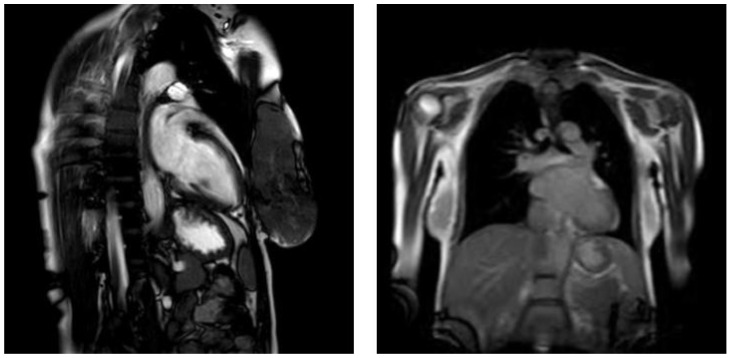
Cardiac MRI of patient reported the enlargement of left ventricle with hypokinesis of the mid-inferior and inferolateral segments one month after birth. LVEF was estimated to be 65%.
